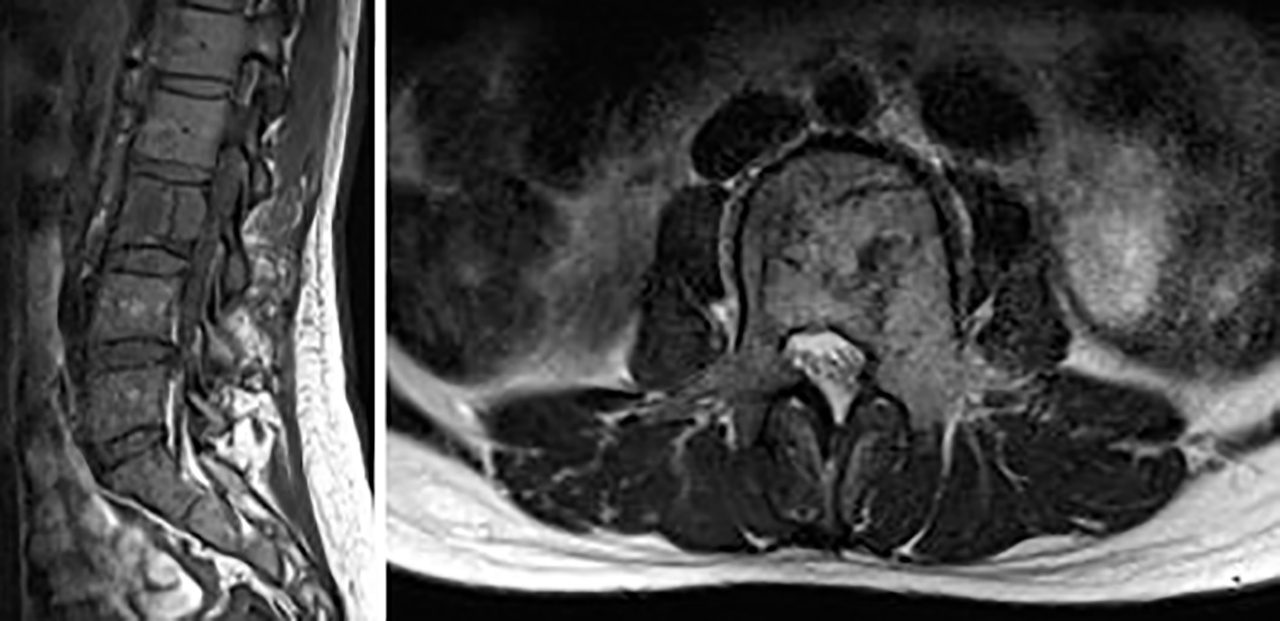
Primary spinal epithelioid hemangioendothelioma (EHE) in a 25-year-old woman. A sagittal image of the lumbar spine reveals the abnormal shape of lumbar 3 vertebral body with a mass in the vertebral body and accessories. MRI showed an inhomogeneous low signal on T_1_-weighted image (T_1_WI) and a high signal on T_2_WI with punctate low-signal foci and accompanying normal discs.
